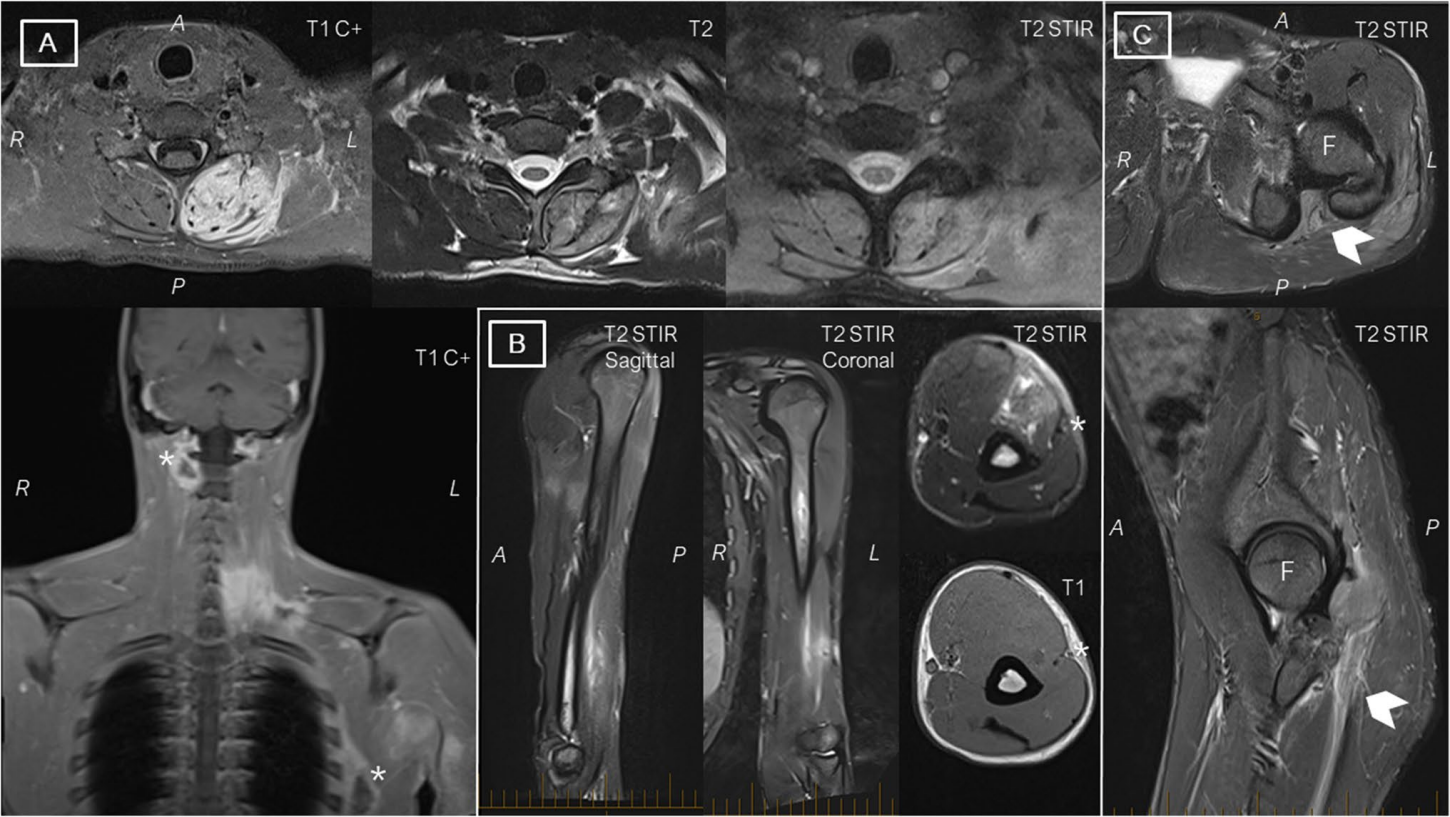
A T2-weighted, T2-STIR, and T1-weighted postcontrast (T1C +) axial images at the level of C7 vertebra show muscle swelling, contrast enhancement, and relatively mild T2-signal change in the left paraspinal muscles. The lack of diffusion restriction and of prominent T2-weighted signal change indicates that this is likely a phlegmon rather than a true abscess. Note additional abscesses identified in the left chest wall and the right paraspinal regions (asterisks) on the T1C + coronal image. B T2 STIR images showing signal change in the brachialis, in close proximity to the neurovascular bundle (asterisk) corresponding to the electrophysiological localization of the patient’s radial neuropathy. C Axial and sagittal T2-STIR images through the left hip demonstrating signal change in the obturator internus and gluteus medius with a T2-STIR hyperintense collection at the musculotendinous junction in the gluteus maximus. Minor compression of the sciatic nerve with extensive signal change consistent with inflammatory neuritis is seen (chevrons). A: anterior; F: femoral head; L: left; R: right; P: posterior
